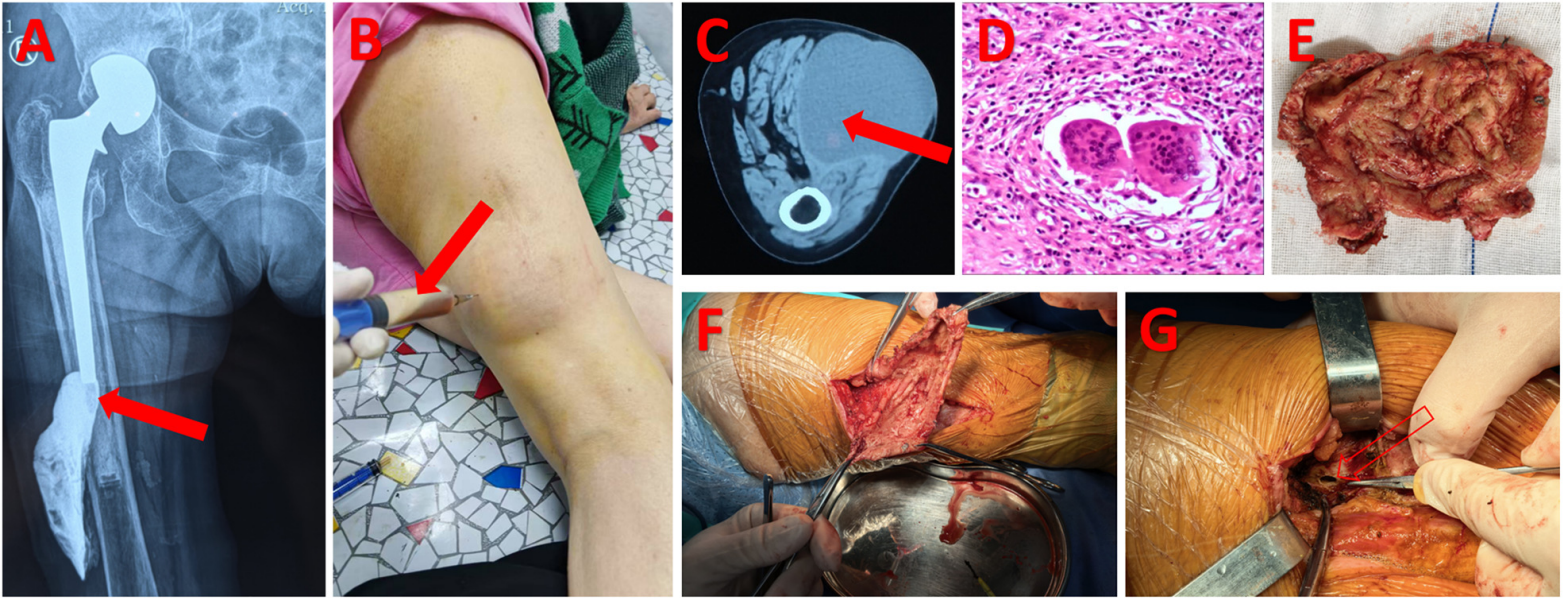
(A) Following the administration of contrast medium into the purulent cavity, DR indicates that the abscess cavity may be connected to the implant [indicated by the solid red arrow in (A)]. (B) Perform a puncture on the liquid within the abscess cavity [indicated by the solid red arrow in (B)]. (C) A massive abscess cavity is indicated by the CT scan [indicated by the solid red arrow in (C)]. (D) Postoperative abscess cavity wall pathology suggests inflammatory cell infiltration. (E,F) Indicate necrosis resembling cheese seen in the abscess cavity wall during surgery. (G) Shows that the abscess cavity was connected to the prosthesis through an oval-shaped defect above the femur during surgery [indicated by the hollow red arrow in (G)].
